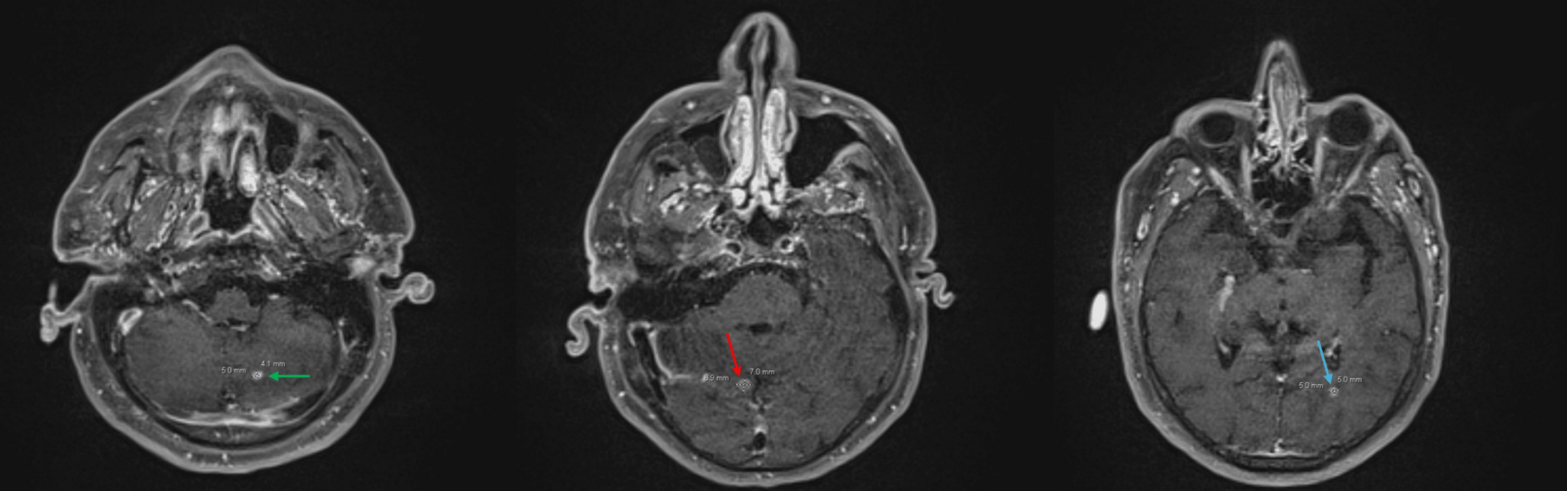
Axial brain MRI showing multiple brain metastases. Post-contrast images of axial brain MRI showing enhancing lesions in the left cerebellar hemisphere (green arrow), right posterior lateral splenic corpus callosum (red arrow), and left occipital lobe (blue arrow).
